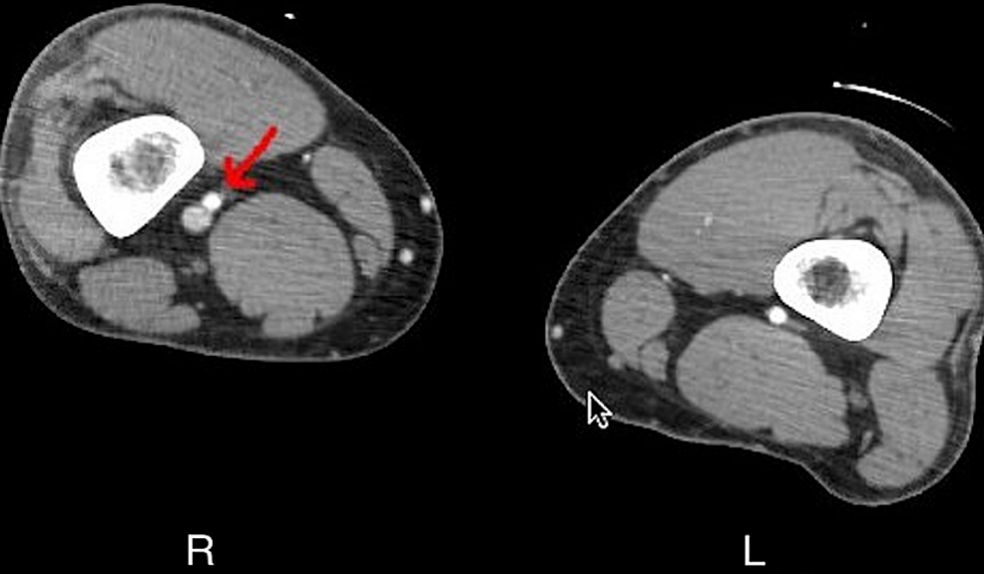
CT angiography with contrast of the lower extremities showing contrast in the vein adjacent to the artery in the right extremity (red arrow)
